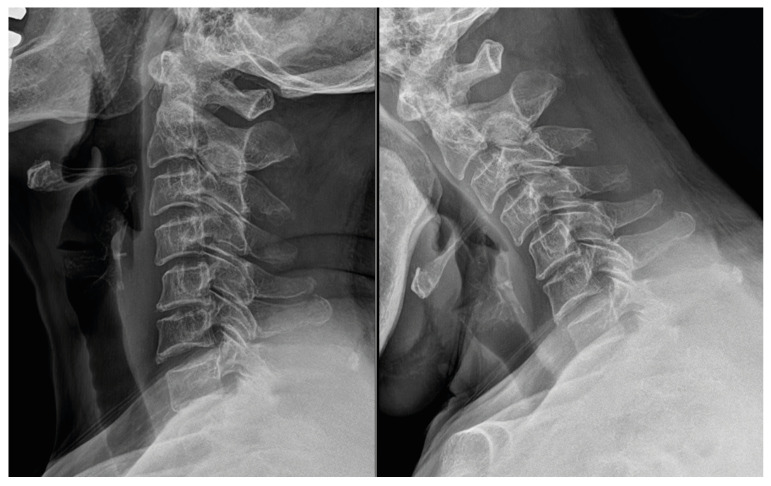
Postoperative plain radiography demonstrating no instability after endoscopic posterior decompression.
